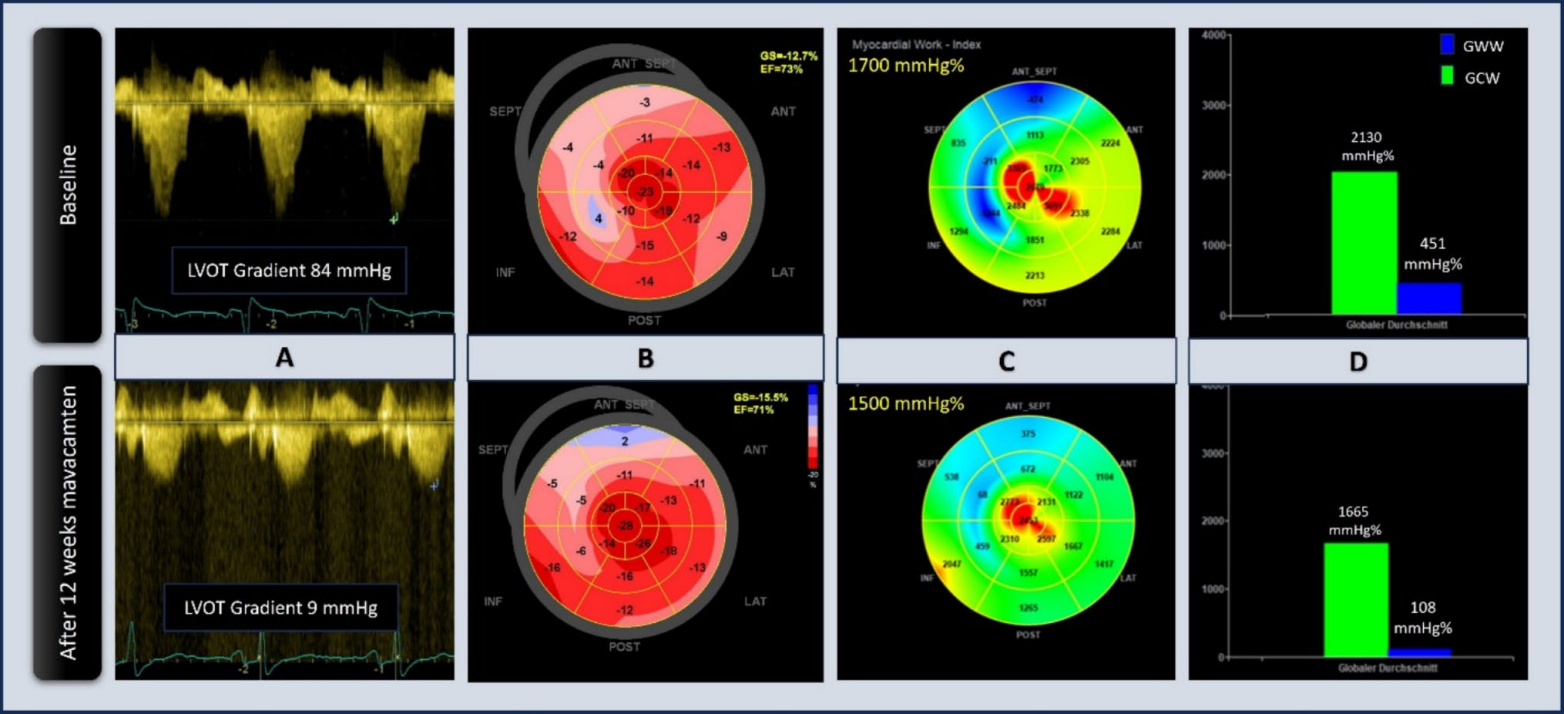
Case example of a patient with obstructive hypertrophic cardiomyopathy demonstrating echocardiographic changes after 12 weeks of Mavacamten therapy. A Resting peak LVOT gradients at baseline and after 12 weeks of Mavacamten therapy. B GLS at baseline and after 12 weeks of Mavacamten therapy. C Myocardial work index (GWI) at baseline and at 12 weeks after Mavacamten. D Global constructive work and global wasted work at baseline and at 12 weeks after Mavacamten. Abbreviations: LVOT, left ventricular outflow tract; GLS, global longitudinal strain; GWI, global work index
